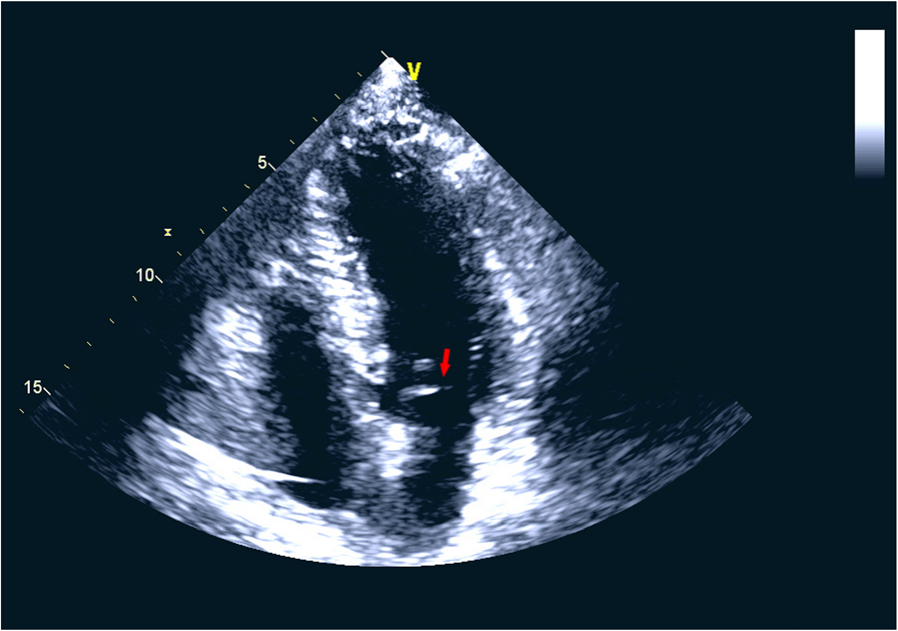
Echocardiography revealed that the artificial aortic valve worked well (red arrow)
